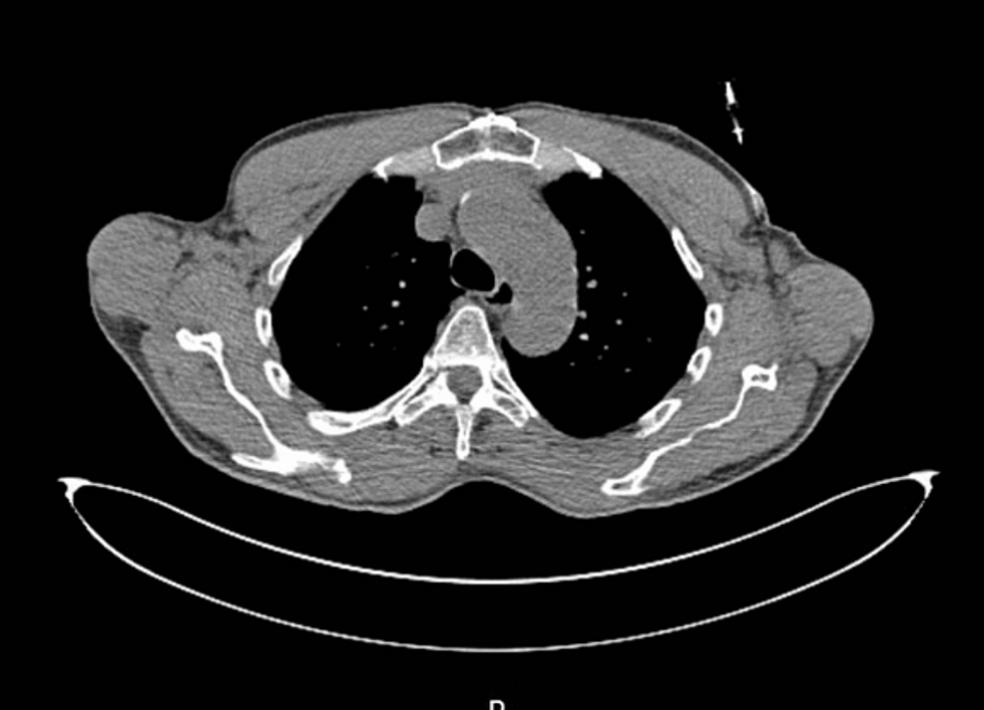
CT-guided lymph node biopsy A transverse section of the upper chest shows needle positioning for biopsy in the left axillary node in May 2021.
